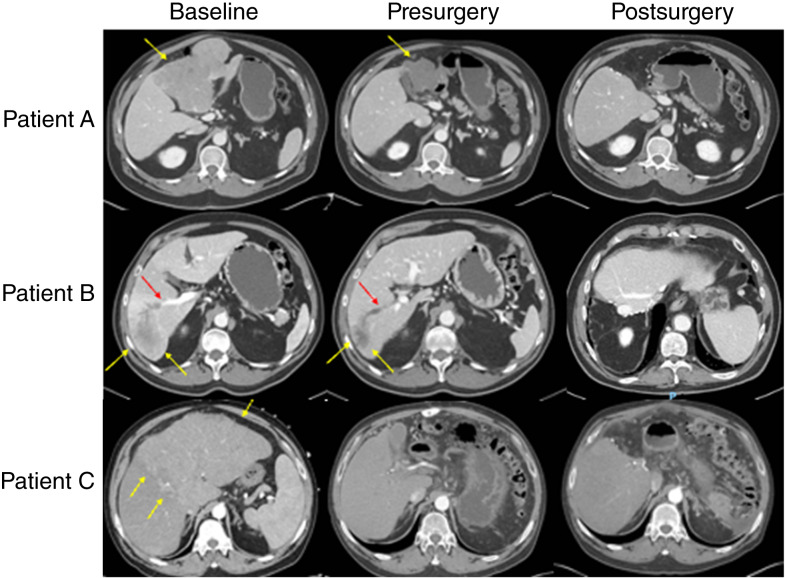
Baseline, presurgery, and postsurgery contrast-enhanced CT images of three BCLC C patients (vascular invasion present) treated with neoadjuvant ICI–based therapy with successful R0 resection. Patient A: CT venous phase imaging showing a large left-liver mass measuring 13.1 cm (yellow arrow) with associated occlusion of the left portal vein (not shown) at baseline. Patient B: CT venous phase imaging showing a 5.3-cm mass with necrosis (yellow arrows) and tumor thrombus within the right portal vein (red arrow); additional multifocal disease (not shown) at baseline. Patient C: CT arterial phase imaging showing large infiltrative lesion involving entirety of the left liver at baseline (yellow arrows) and associated left portal vein invasion (not shown), with complete radiographic response to ICI therapy prior to surgery.
